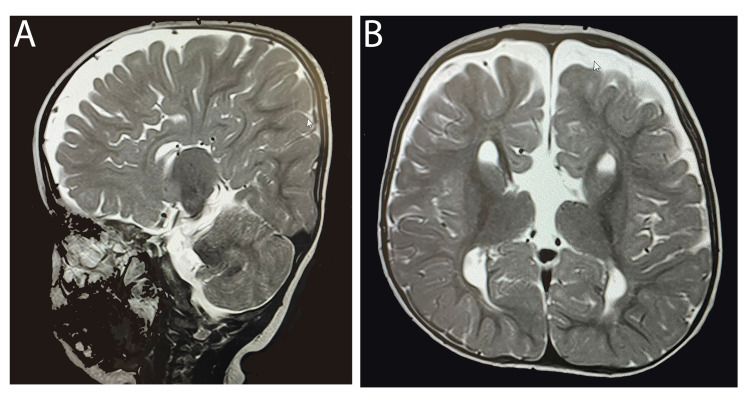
MRI of Case 1: (A) sagittal section (B) coronal section showing complete corpus callosum agenesis
